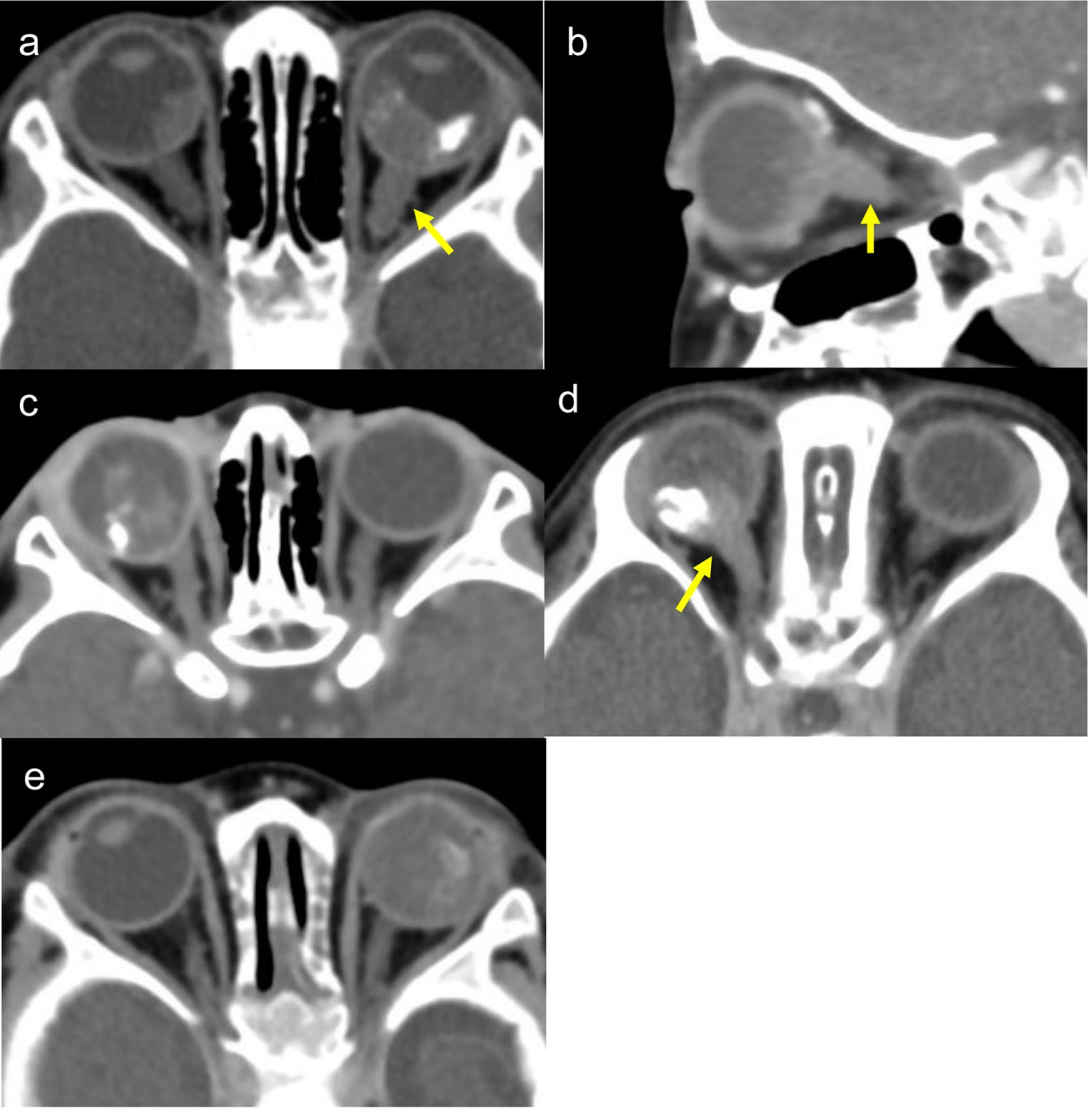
Contrast-enhanced computed tomography (CT) images of Cases 1–5 (a-e). ON swelling and contrast enhancement indicating ON invasion (arrows) are seen. There is no evidence of ON invasion (c, e)
